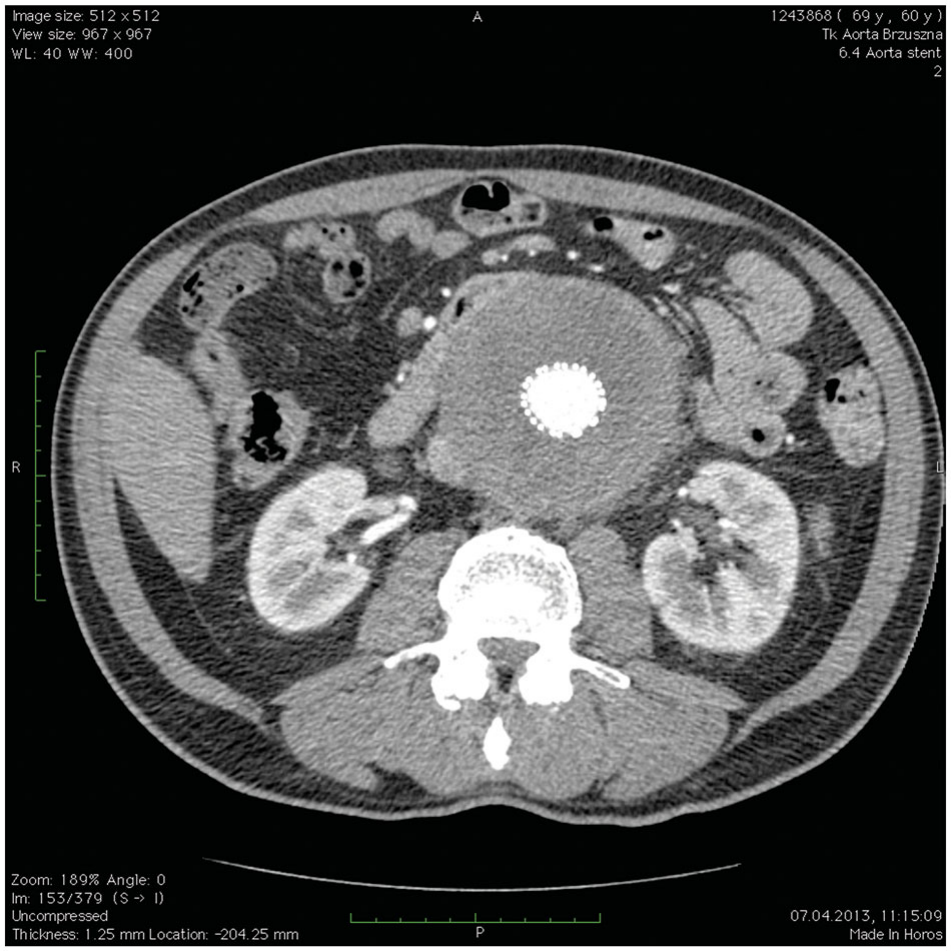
CT scan of subjects with vascular subtype of retroperitoneal fibrosis. Scan shows an aortic inflammatory aneurysm after endovascular stent insertion.
